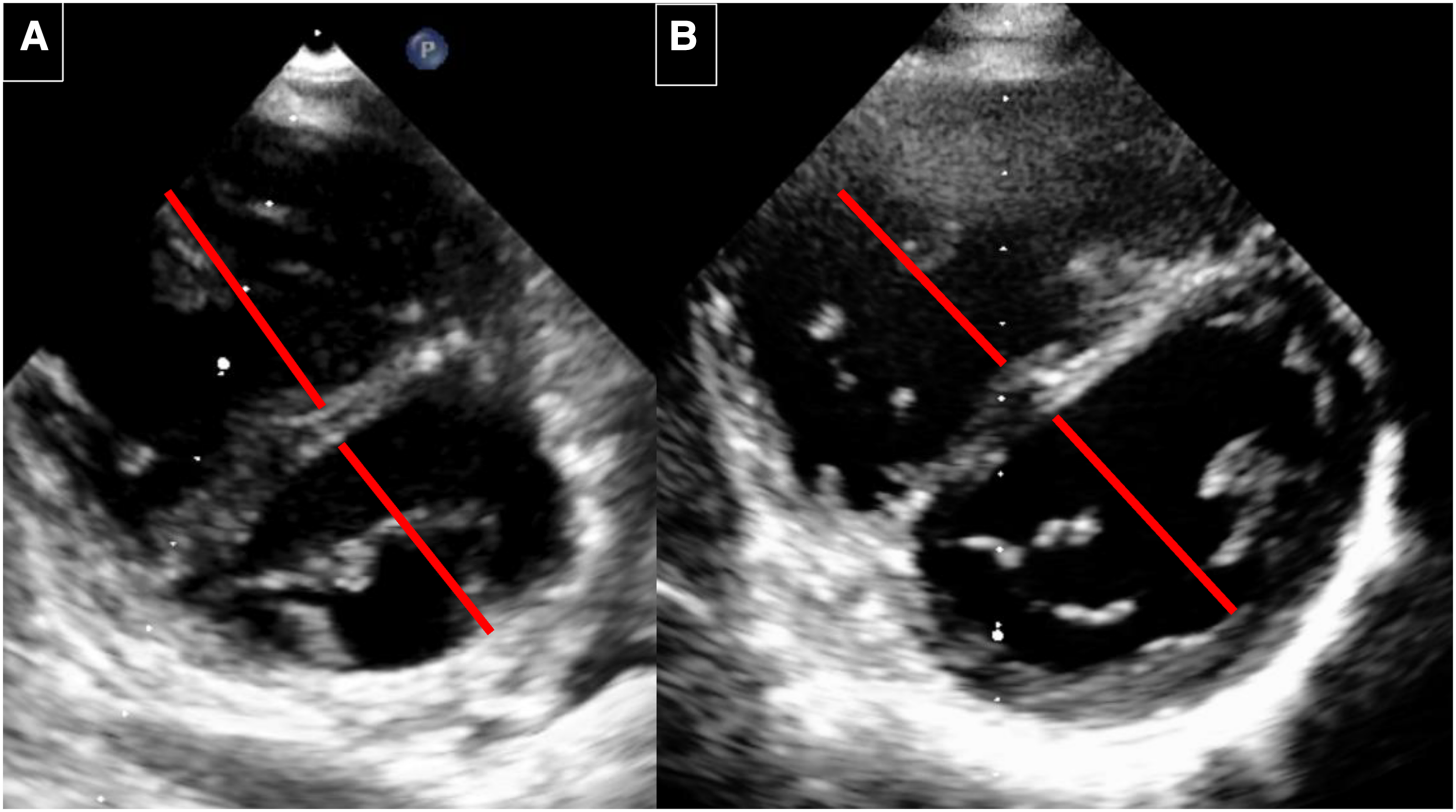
Echocardiography before and 6 years after implantation. (A) Before implantation: RV with septal flattening before AFR implantation. The RV/LV diameter ratio is 1: 0.87. (B) 6 years after AFR implantation. RV with decrease of the RV volume and improved LV filling. The RV/LV ratio is 0.87/1.
